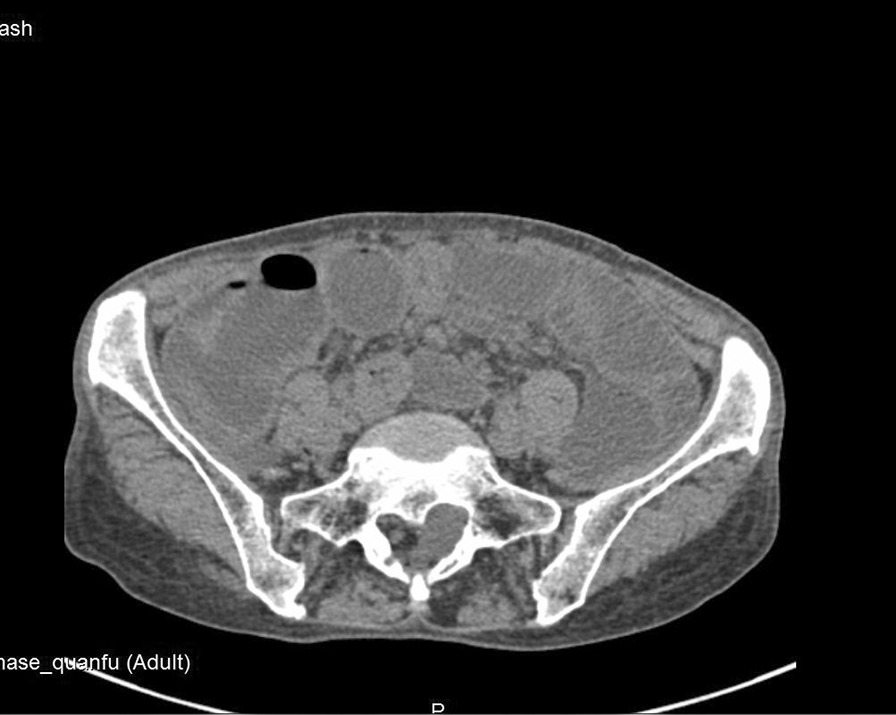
No intussusception was found on abdominal CT before operation
